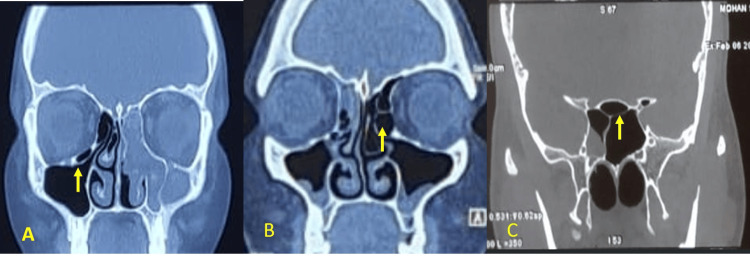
Coronal CT images of paranasal sinus showing types of air cells A: Haller cell; B: agger nasi cell; C: Onodi cell CT: computed tomography
